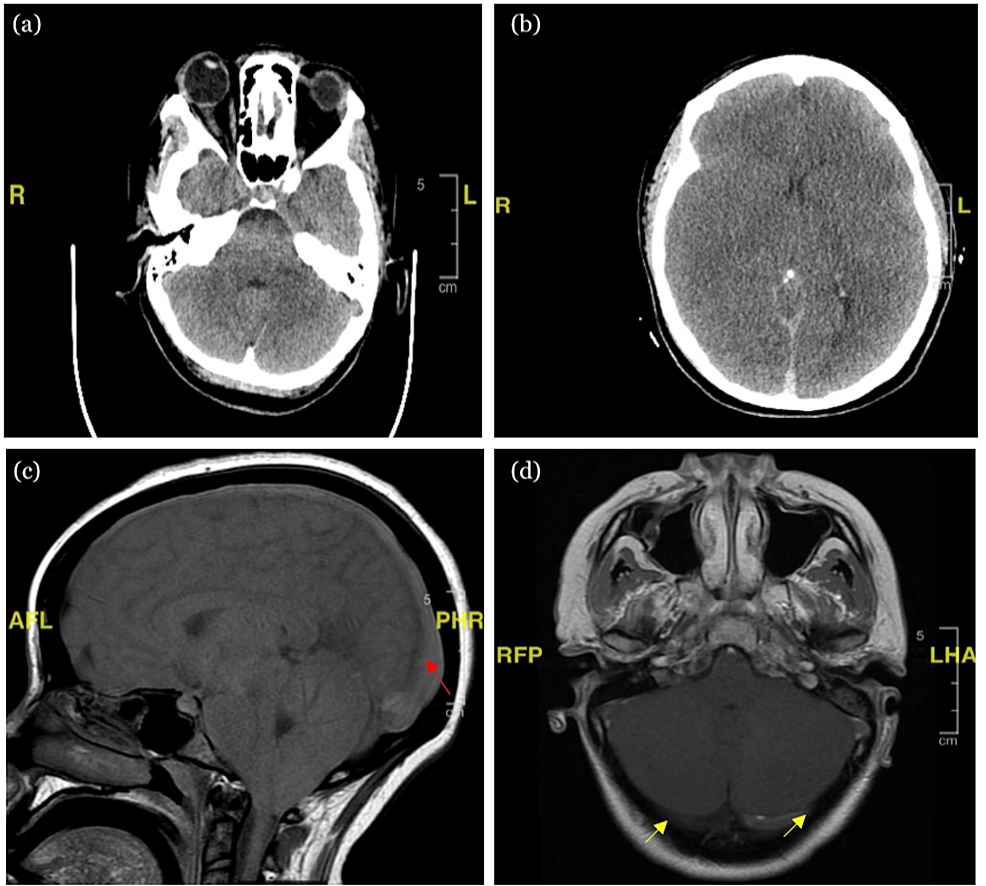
Imaging of the brain (A) Initial non-contrast-enhanced CT of the brain with no acute findings. (B) CT of the brain venography demonstrating diffuse cerebral edema. MRI of the brain (C) sagittal view (red arrow) and (D) axial view (yellow arrows) showing evidence of sinus thromboses and lack of blood flow.
